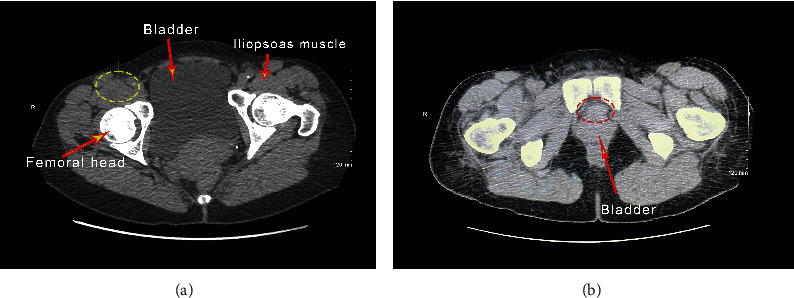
CT scan of the abdomen and pelvis demonstrating a psoas abscess ((a) yellow circle) and a 20 × 15 × 14 mm soft tissue density in the inferior posterior left paramedian bladder wall ((b) red circle).
